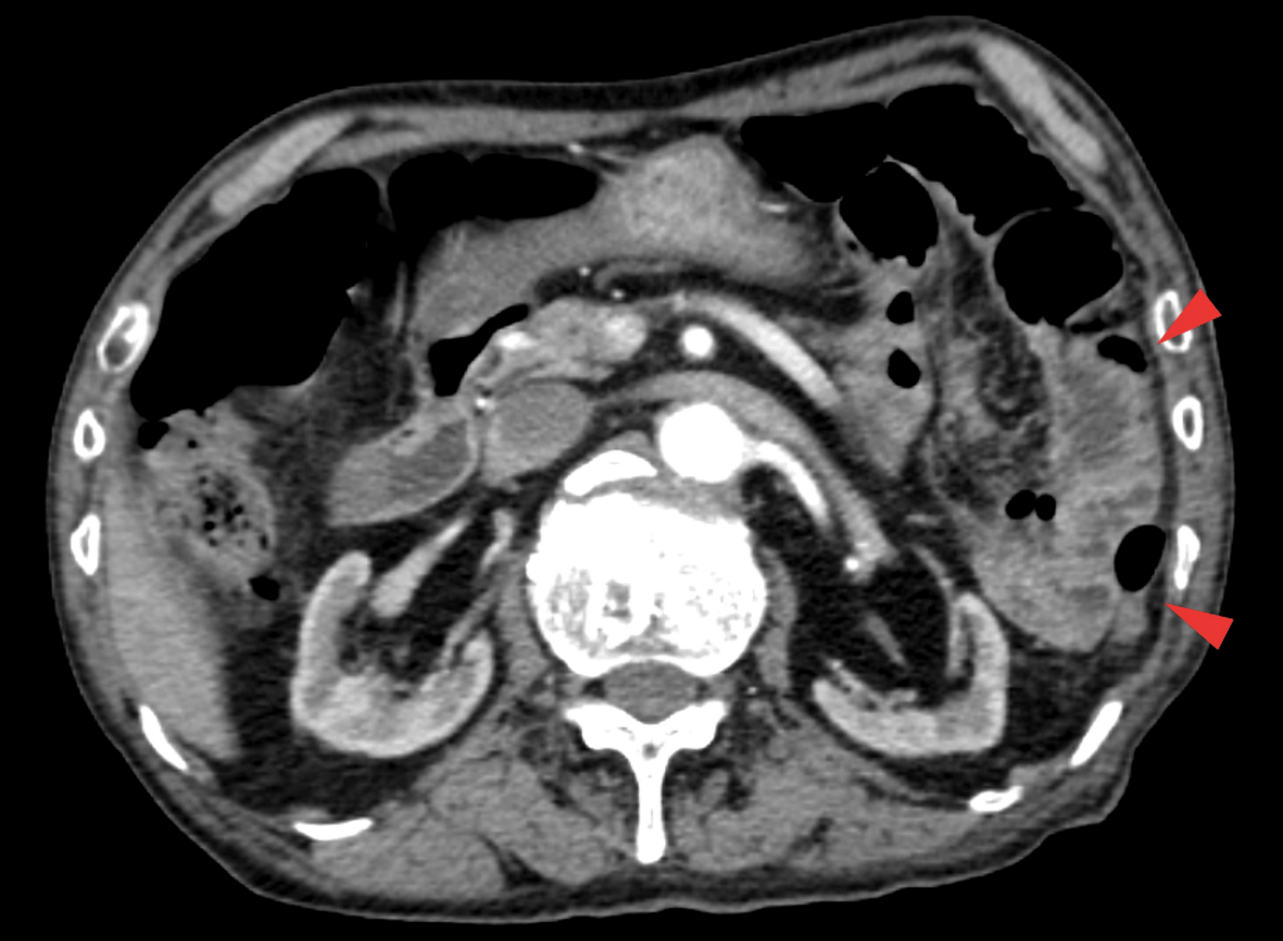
Contrast-enhanced abdominal CT (axial view) Thickened wall of the left colon and surrounding fat stranding (arrowheads) are evident.
